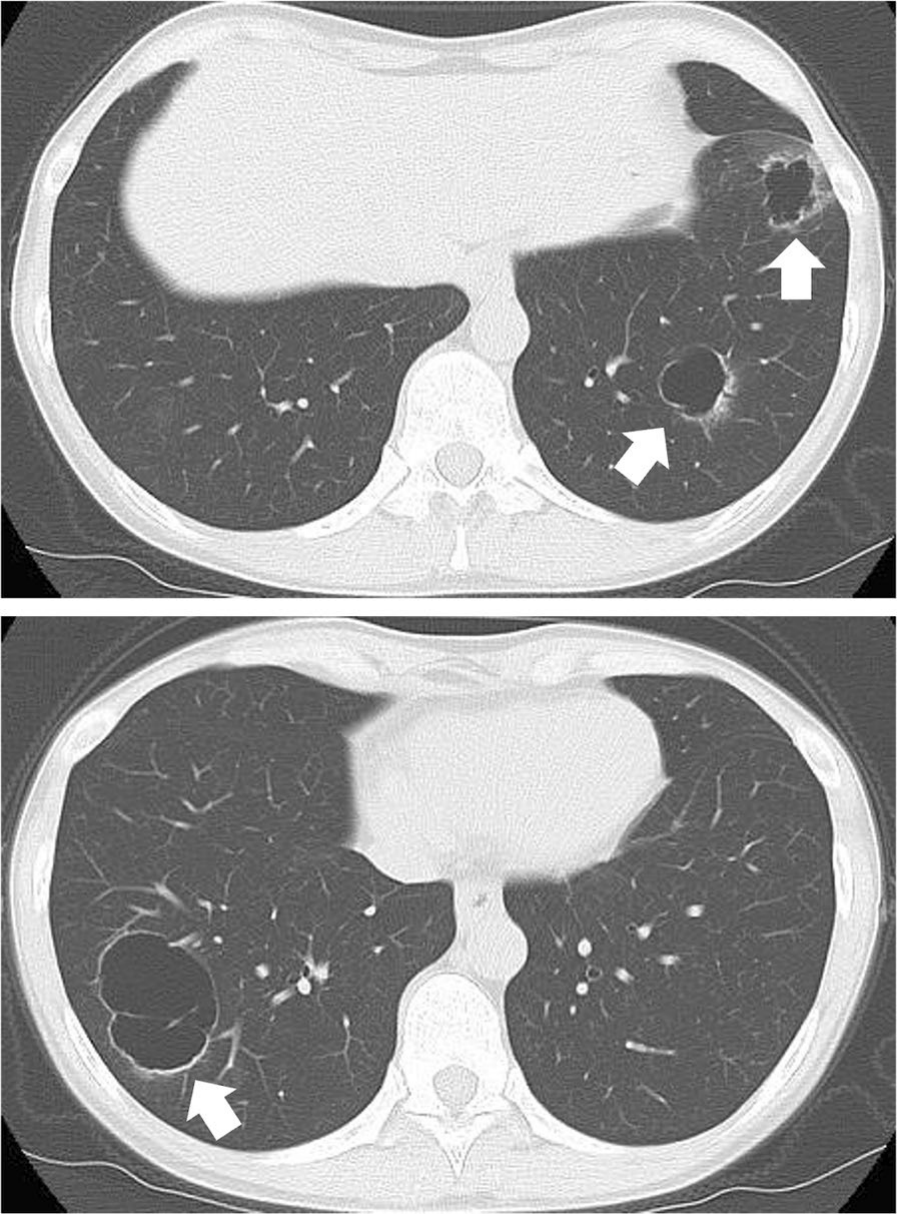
Plain chest computed tomography scans 3 years and 6 months after the initial surgery. Multiple cystic lesions were found in both lungs (white arrow)
